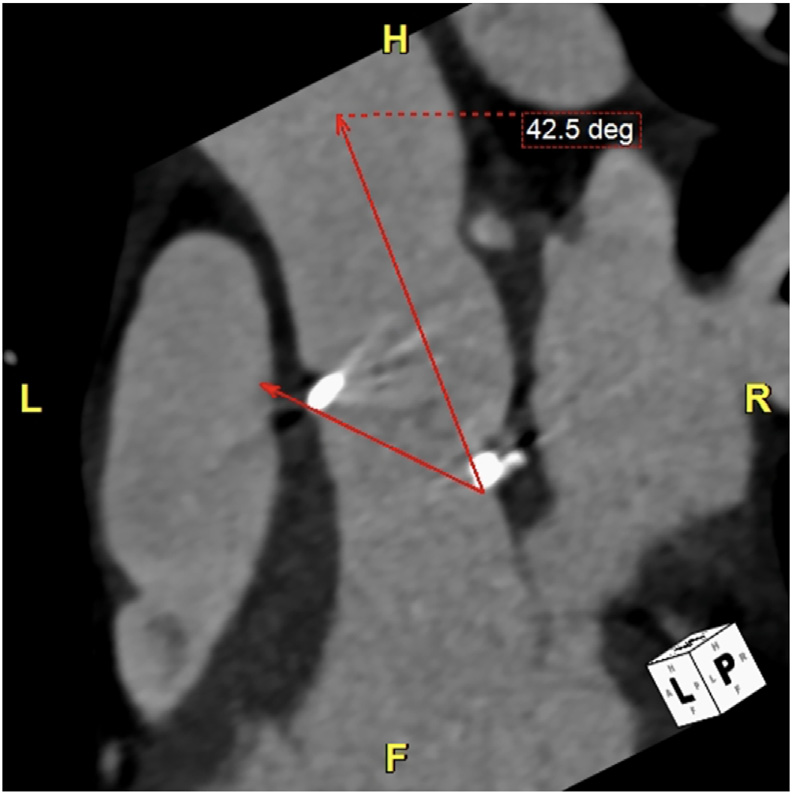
Computed tomography scan showing the angle between the aorta and valve to be 42.5 degrees. (F, foot.; H, head; L, left; R, right.)
